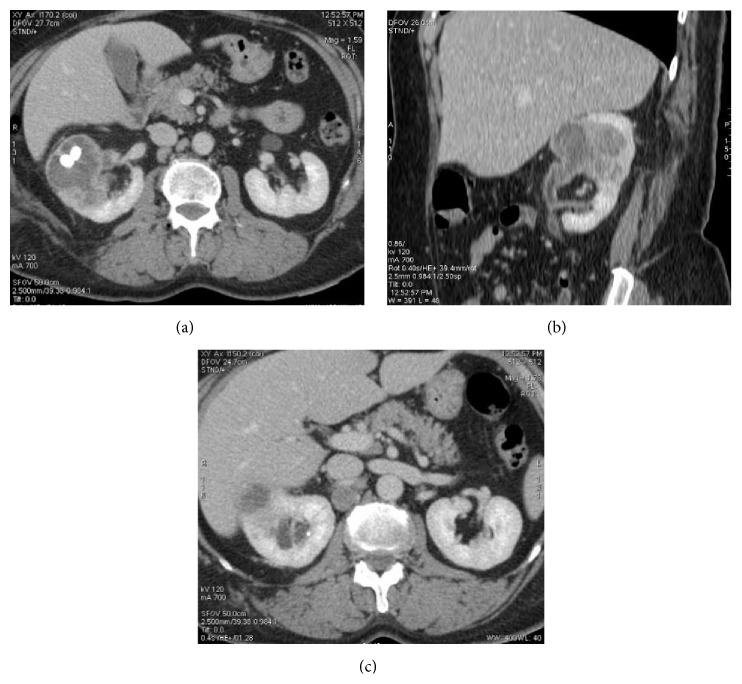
Preoperative CT scan. (a) Axial and (b) coronal enhanced CT scan showing a mixed solid and cystic mass at the middle third of the right kidney containing two centimetric renal calculi. (c) Axial enhanced CT scan showing a centimetric lymph node in the retrocaval region.
